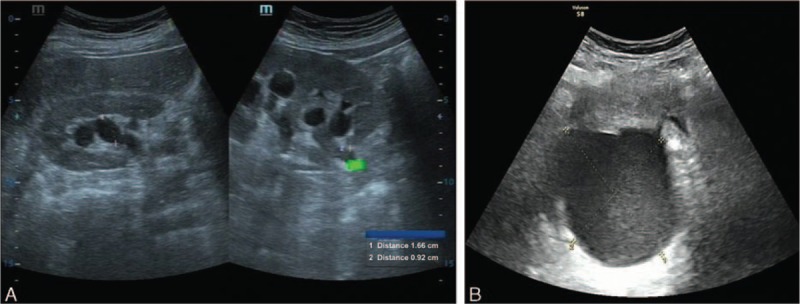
(A) Urinary ultrasound showing right-sided hydronephrosis and irregular upper section of the right ureter. (B) Gynecological ultrasound showing a cystic mass (8.8 × 7.5 × 8.6 cm) in the right rear uterus. The mass was grossly well circumscribed.
